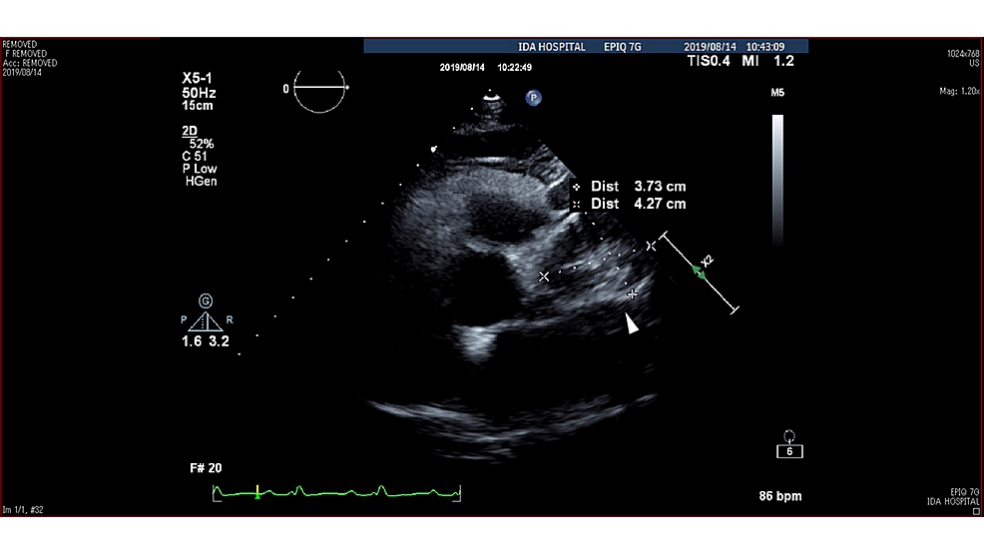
Transthoracic echocardiography Transthoracic echocardiography shows an elliptical hypoechoic lesion (white arrow) with a diameter of 43x37 mm between the PA and left coronary artery. A thrombus-like region of moderate echogenicity is present inside the lesion. PA: pulmonary artery
